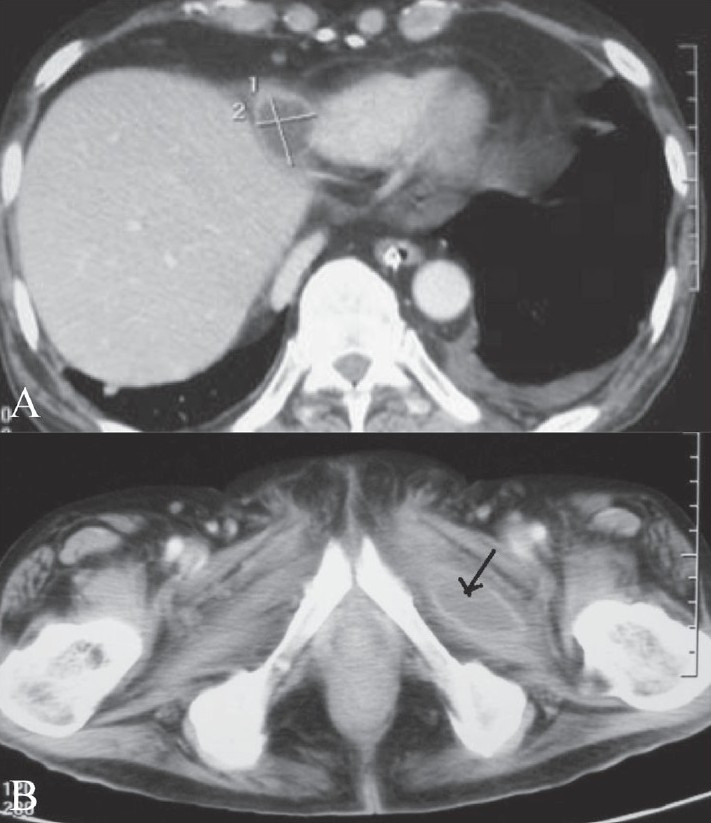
Along with an abscess in the right paracardiac region (A) CT scan of the abdomen shows an abscess (arrow) in the left obturator externus muscle (B)
